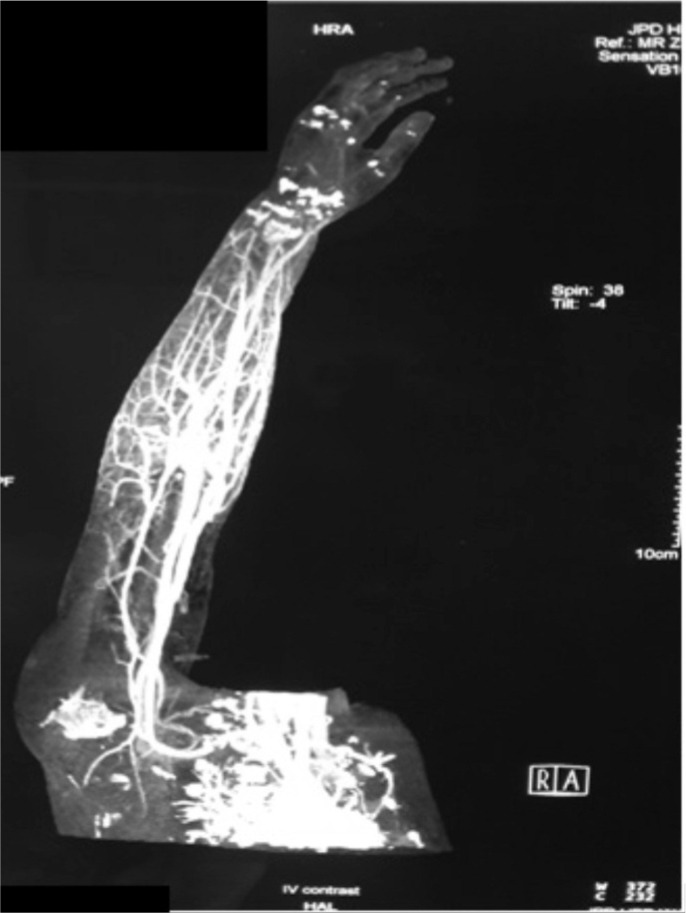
CT Angiogram right upper limb.
